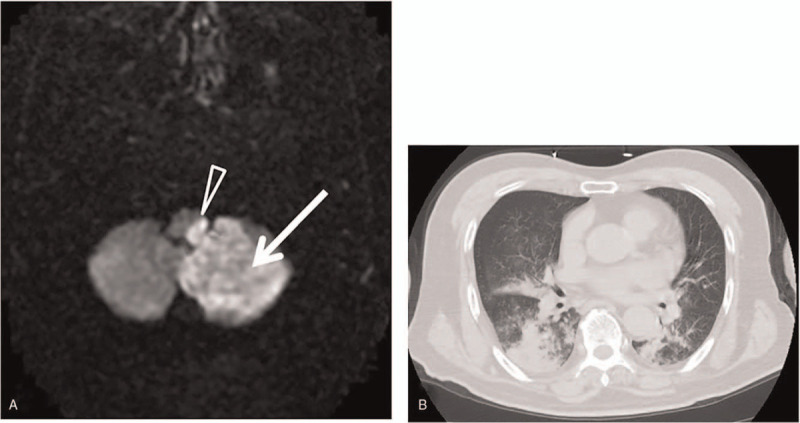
(A) MR diffusion weighted imaging suggests the acute medullary infarction in left-side cerebellar (white arrow) and medullary (white sharp triangle). (B) Sagittal plane CT of chest showed multiple inflammatory patchy shadows in both lungs.
